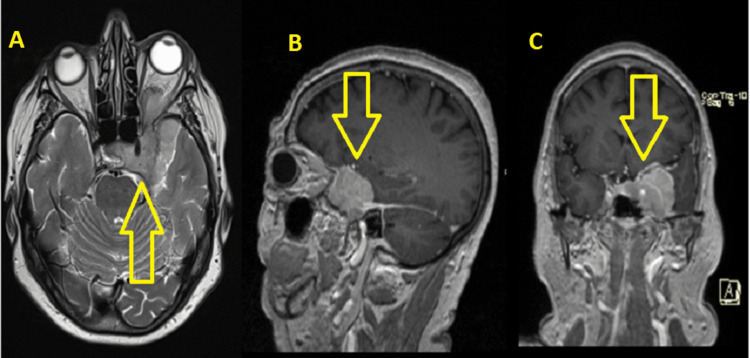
Preoperative MRI. Radiological findings of the tumor. A - Axial plane. B - Sagittal plane. C - Coronal plane.
